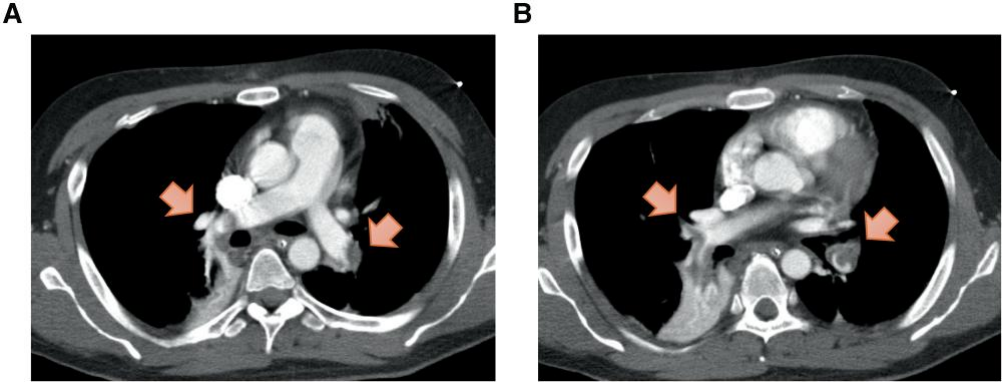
Findings on computed tomography images obtained on hospital Day 13. (*A*, *B*) Images showing newly developed thrombi in the right middle lobe and left lower lobe branches of the pulmonary artery (arrows).
